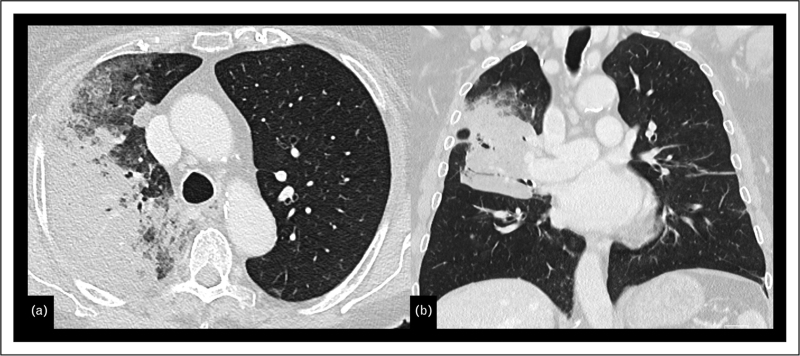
Transverse (a) and coronal (b) CT image reconstructions of the lung show a consolidation with air-bronchogram in the right upper and middle lobes. As the tumor is located in two different ipsilateral lobes and due to the tumor size, it is categorized as T4.
